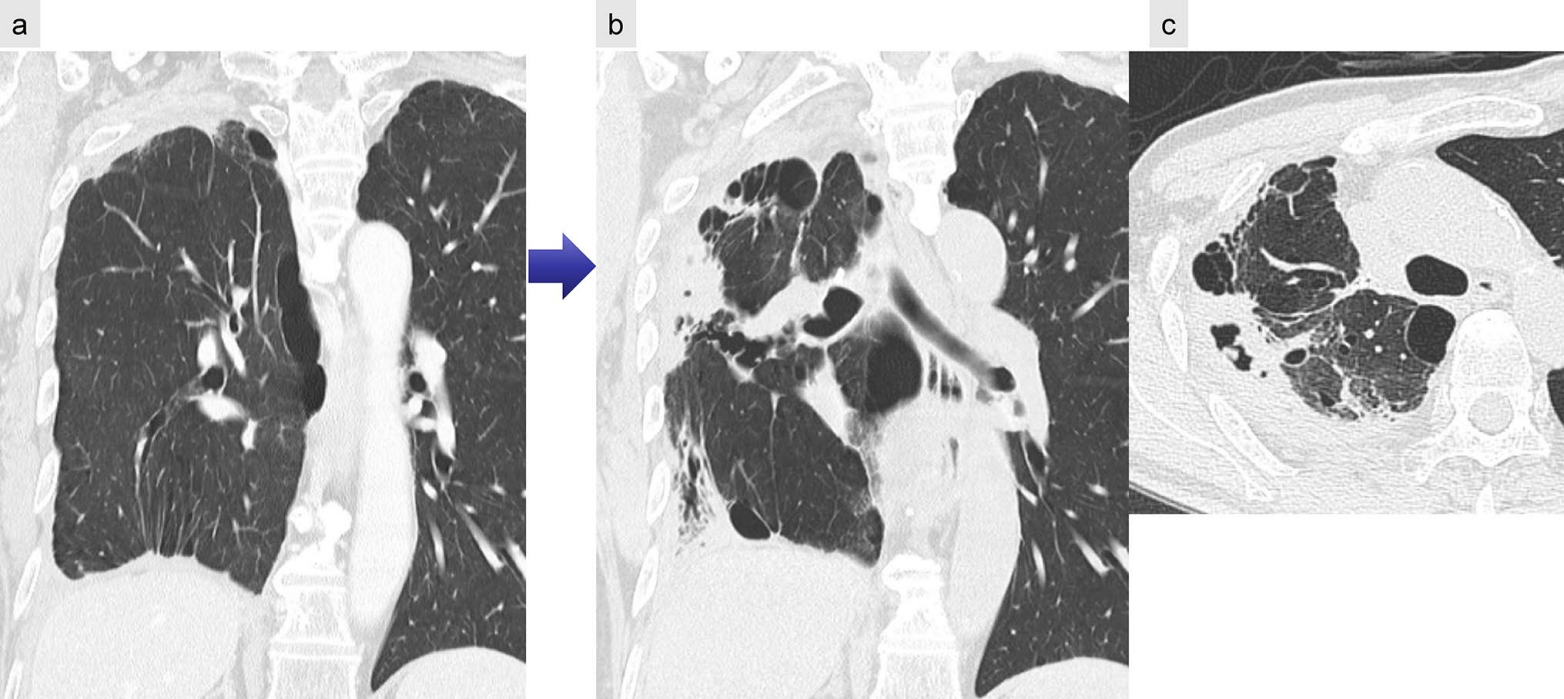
Unilateral pleuroparenchymal fibroelastosis. Seven years after right upper lobectomy, coronal CT images at postoperative year 1 (a) and year 7 (b) show progressive thickening of the apical cap, cystic changes in the right upper and middle lung fields, volume loss, and thoracic cage contraction. Axial CT at year 7 (c) reveals a nodule suspected to be a fungal ball within a sub-pleural cyst, leading to a diagnosis of aspergillosis
